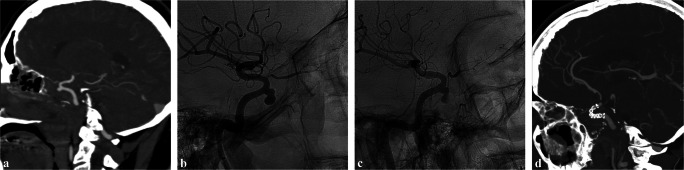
Case #5 was a 54-year-old male affected by poorly differentiated sphenoid sinus squamous cell carcinoma (pT4bN0M0), extended bilaterally to cavernous sinuses and with a 360° encasement of right ICA, previously submitted to chemotherapy (2 cycles of cisplatin and paclitaxel) and intensity-modulated proton beam therapy (70 Gy). The patient experienced massive epistaxis, investigated with diagnostic imaging, including CTA and DSA, which showed cavernous ICA PSA. The bleeding was controlled by FDS placement. a and b Pre-procedural CTA (a) and DSA (b) showing anterior genu right ICA cavernous segment PSA. c Intra-procedural DSA showing FDS correct placement. d CTA at 1 month demonstrating PSA resolution
